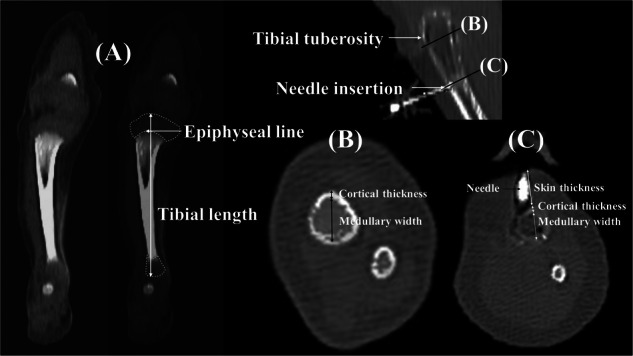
Radiological measurements from computed tomography scan images of the tibial bone. A Longitudinal view demonstrates measurement of tibial length. B Cross-sectional view measurements at the widest medullary cavity of the proximal tibial bones. C Cross-sectional view measurements at the intraosseous needle insertions.
